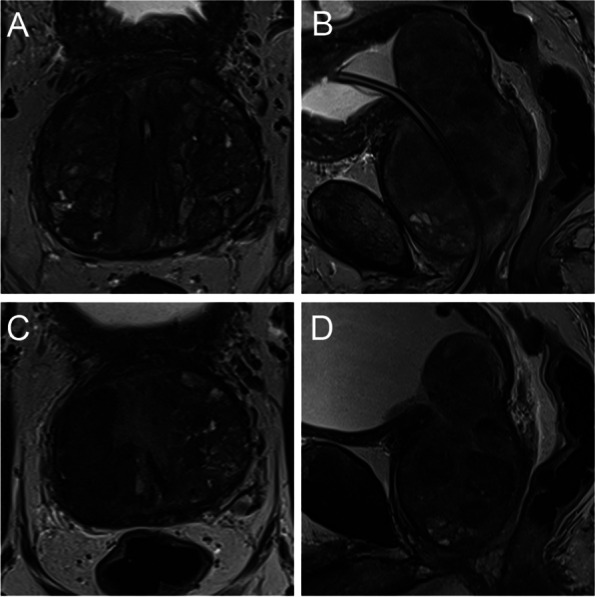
Wasserman type 5, modified BPH classification 3 (biurethral); A T2-weighted axial baseline B T2-weighted sagittal baseline; bilateral TZ hyperplasia with dominantly pedunculated pattern; C T2-weighted axial follow-up D T2-weighted sagittal follow-up. Volume reduction of 293 ml to 175 ml (40%); IPSS 17 to 2 (88%)
